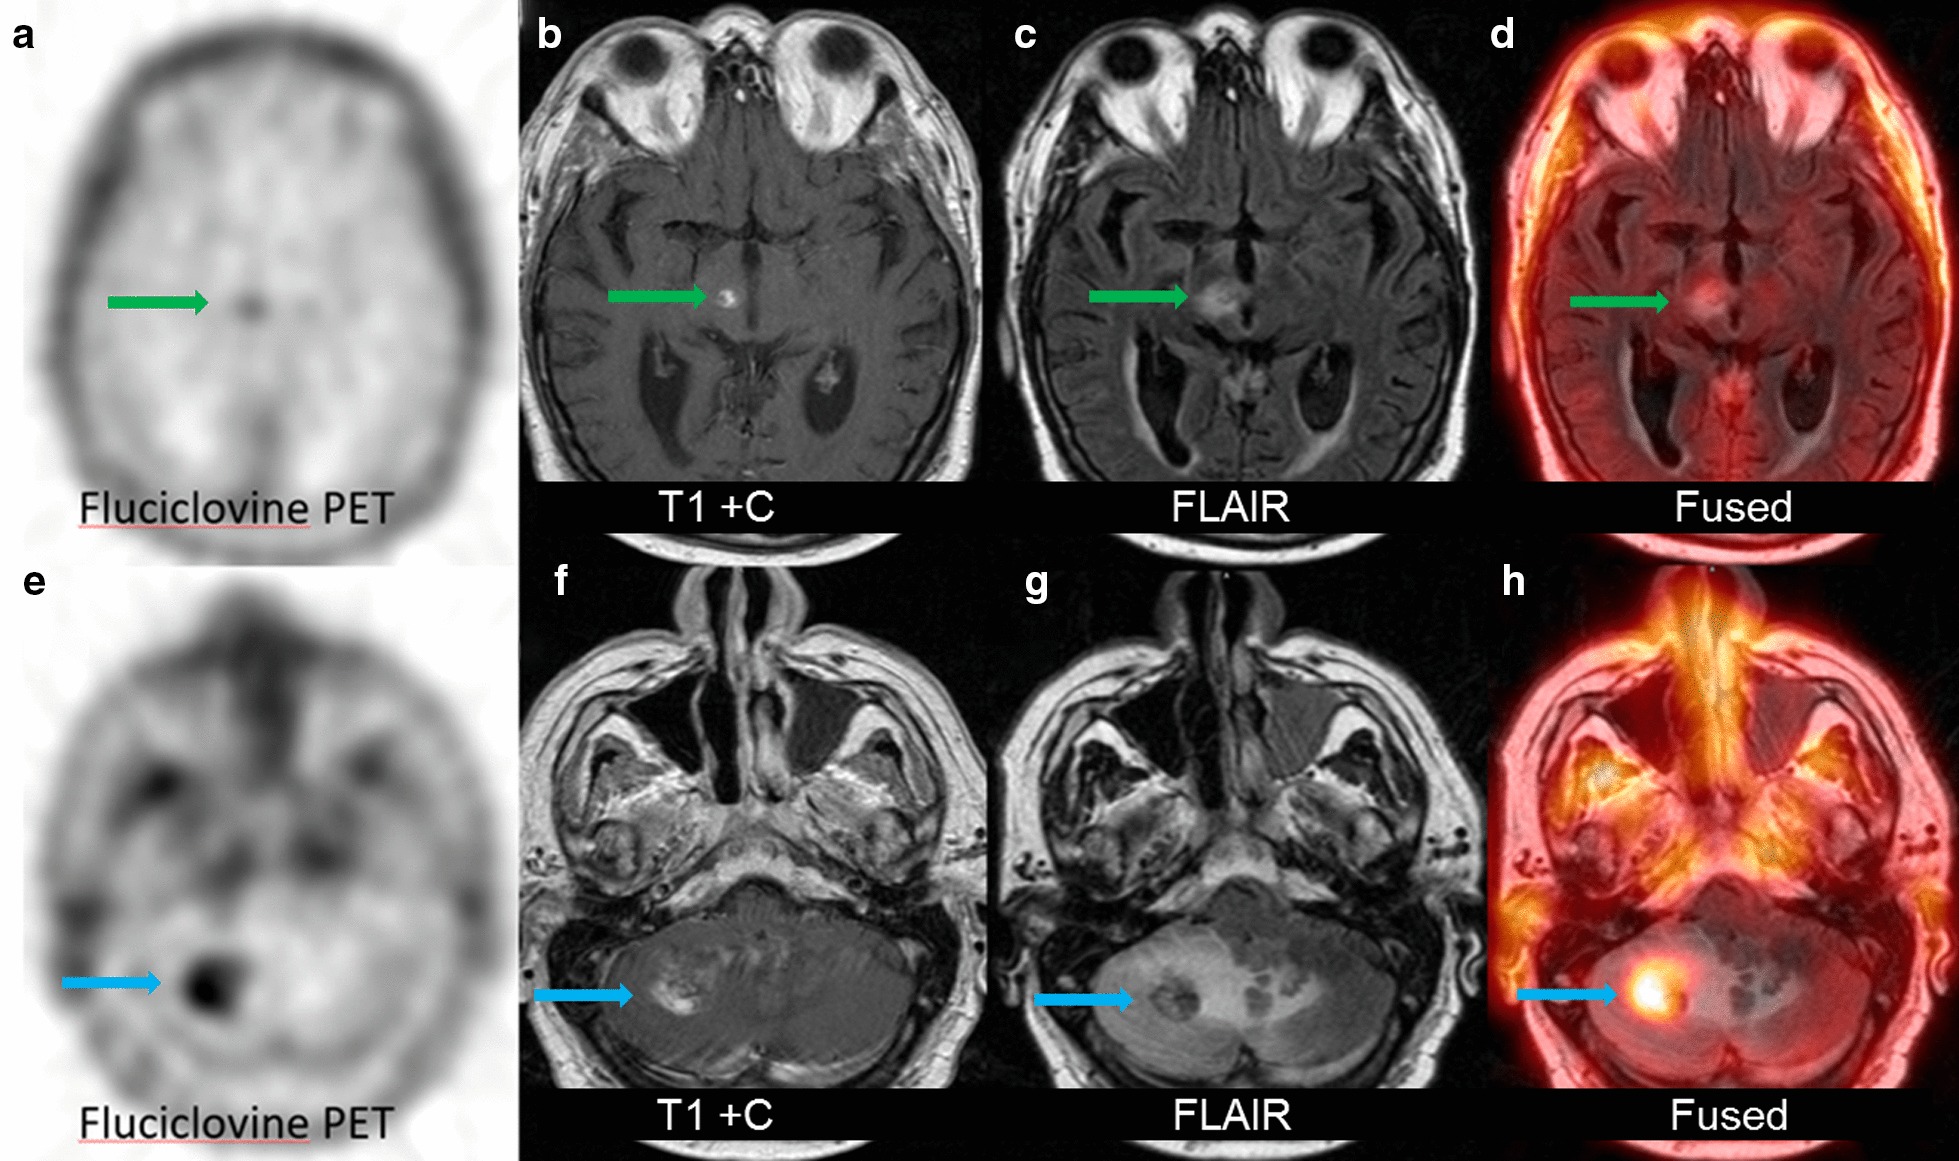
A 54-year-old patient with metastatic renal cell carcinoma and prior stereotactic radiosurgery. Follow-up MRI demonstrated progressively enhancing brain lesions suspicious for recurrent disease. Top panel demonstrates that a right thalamic lesion (green arrow) had low fluciclovine uptake (SUV_max_ of 1.0) as seen on transaxial PET (a), corresponding T1 + contrast (b), focal FLAIR hyperintensity (c), and fused FLAIR and PET (d). This lesion did not increase in size on follow-up MRI and was considered consistent with radiation necrosis. A right cerebellar lesion (blue arrow) in the same patient had high fluciclovine uptake (SUV_max_ of 5.3) on transaxial PET (e) and corresponding T1 + contrast (f) FLAIR hyperintensity (g) and fused FLAIR and PET (h). The right cerebellar lesion was found to be recurrent metastatic disease upon resection
